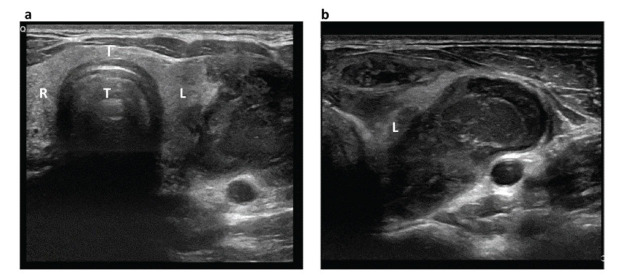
Thyroid ultrasound after one week of antibiotic treatment, in transversal section (a), with focus on the left lobe (b). An inhomogeneous area of colliquate appearance can be observed in the left thyroid lobe. T=trachea, I: thyroid isthmus, L=left thyroid lobe; R= right thyroid lobe.
